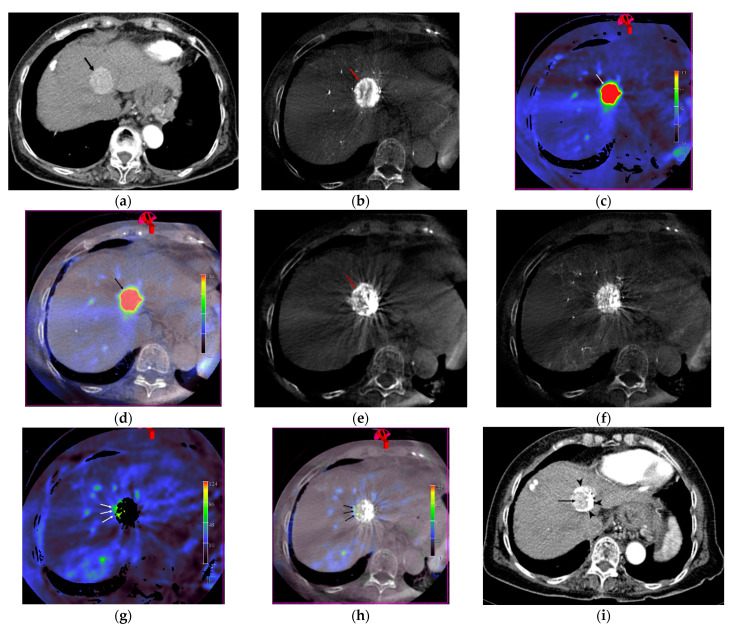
A 72-year-old woman with advanced liver cirrhosis and hepatocellular carcinoma (HCC) in segment 4 of the liver. She underwent transarterial chemoembolization. (a) An approximately 3.0 × 2.6-cm enhancing mass (arrow) is noted in segment 4 of the liver in the arterial phase of contrast-enhanced dynamic computed tomography (CT) of the liver. (b) A contrast-enhancing mass (arrow) is demonstrated on enhanced cone-beam CT. (c,d) The mass (arrow in c, d) shows increased perfusion, seen in red, on cone-beam CT-based perfusion-mapping; pure perfusion imaging (c) and fusion image of perfusion image and pre-transarterial chemoembolization (TACE) unenhanced CBCT image (d). (e) Compact ethiodized oil deposition is noted at the previously noted viable tumor (arrow) on unenhanced cone-beam CT performing after chemoembolization. (f) A viable tumor is not clearly observed on enhanced cone-beam CT performing after chemoembolization. (g,h) A focal, nodular, increased perfusion area, seen in yellow and light green color (arrow in g, h), suspicious for residual tumor, is demonstrated at the medial aspect of the mass by ethiodized oil deposition on cone-beam CT-based liver-perfusion-mapping performed after chemoembolization; pure perfusion imaging (g) and fusion image of perfusion image and post-TACE unenhanced cone beam computed tomography (CBCT) image (h). (i) CT image obtained 3 months after transarterial chemoembolization shows an enhancing portion (arrow) at the medial aspect of the treated HCC with ethiodized oil deposition (arrowhead). This enhancing portion (arrow) was presumed to be a viable tumor portion.
